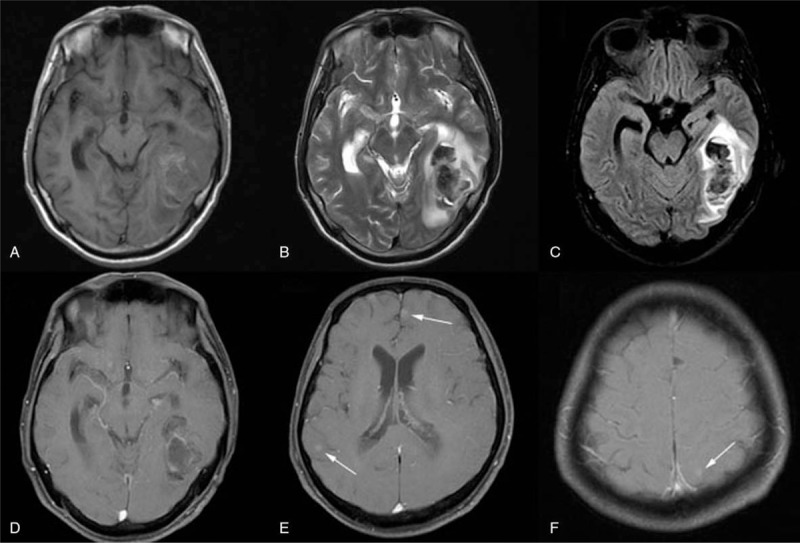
Magnetic resonance imaging enhancement of brain. A–D showed a lesion in the left temporal occipital lobe and bilateral ventricle hemorrhage. E and F, Gadolinium-enhanced T1-weighted image. It shows several enhanced spots on the left frontal, parietal, and right temporal lobes.
